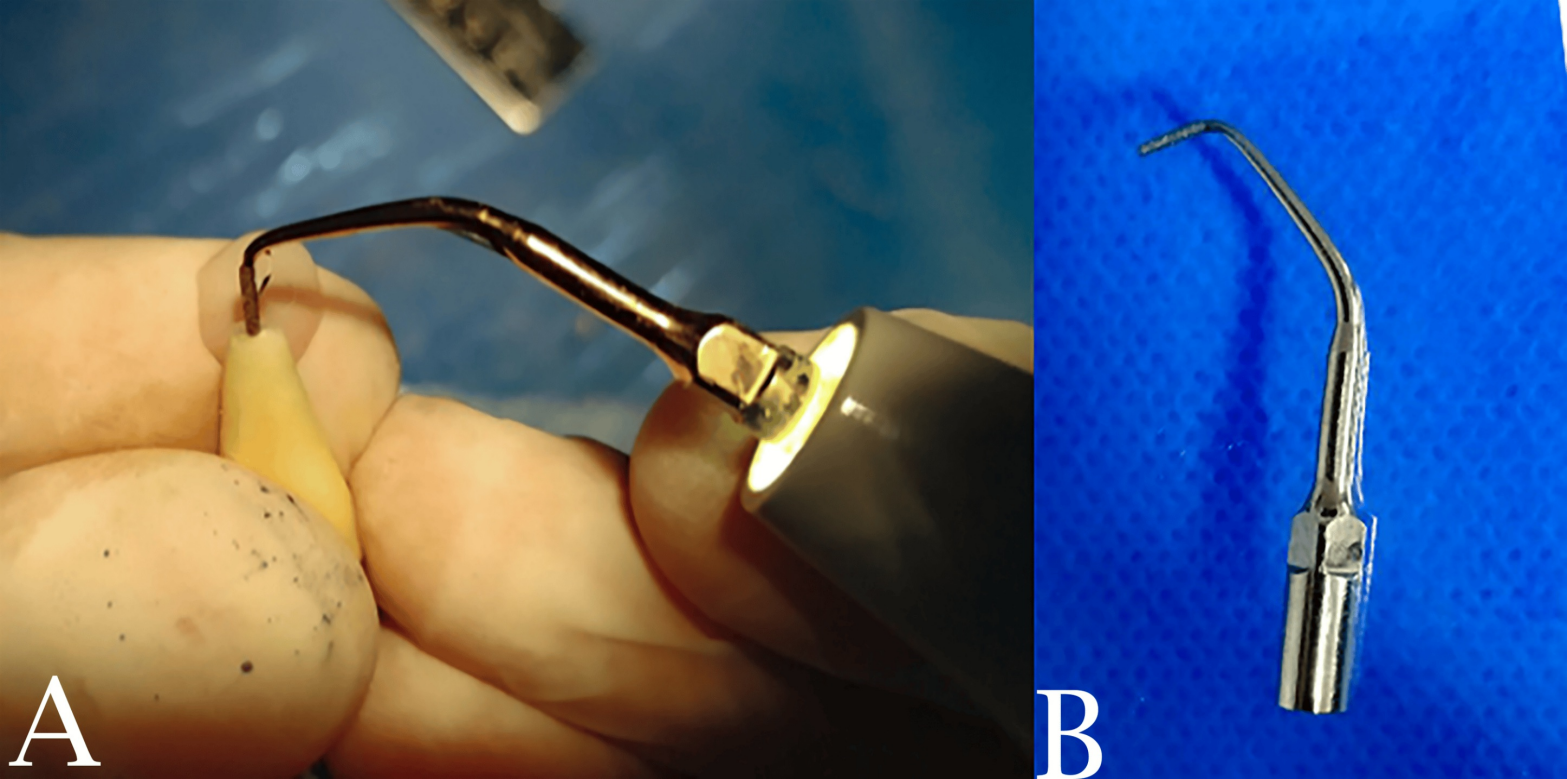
Retrograde cavity preparation using the ultrasonic system. A: Cavity preparation. B: The ultrasonic tip E10D.
